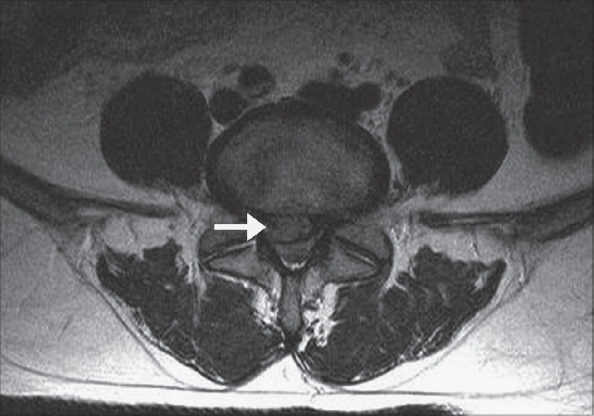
Axial MR image of a patient in the primary discectomy category - MRI showing extruded disc (arrow)
